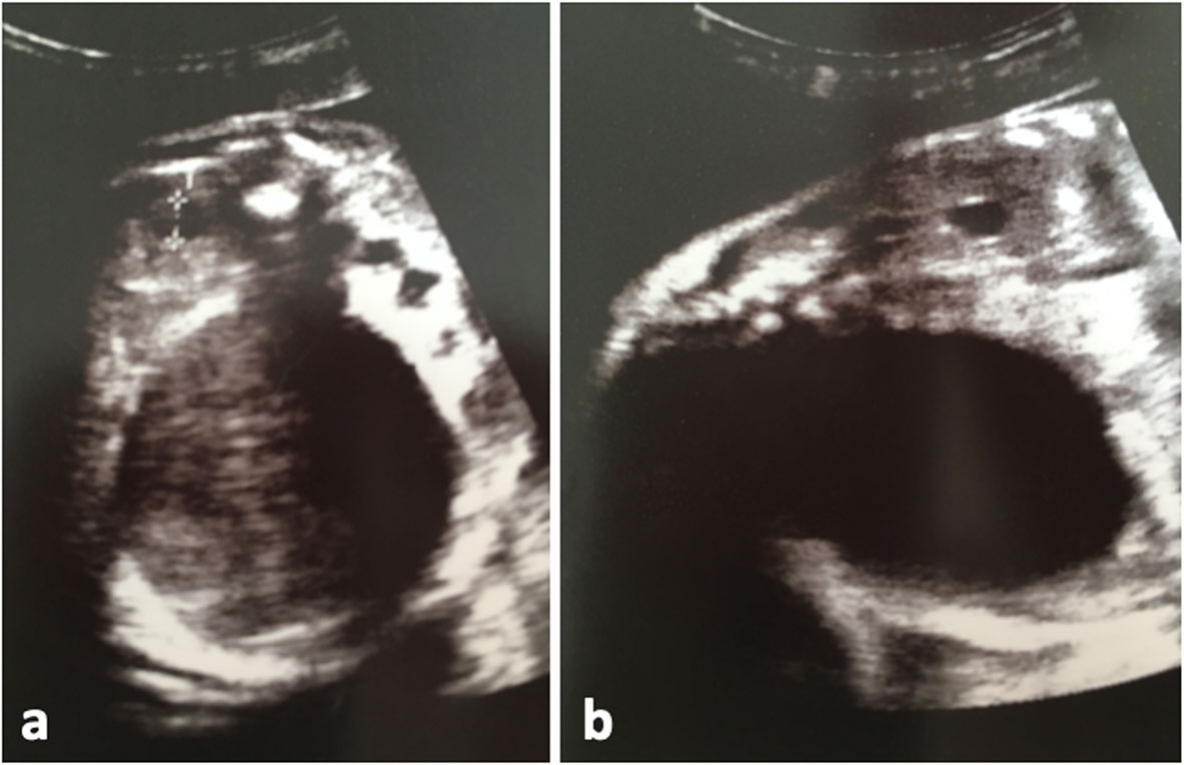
Fetal ultrasound at 28 weeks and 5 days. a Cross-section and (b) longitudinal: megacystis with renal impact. Presence of bilateral pyelocaliceal dilatation
